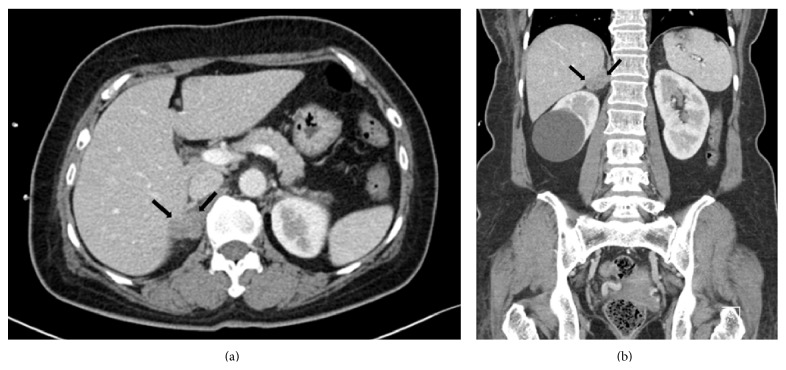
Axial (a) and coronal (b) abdominal computed tomography images during the late arterial phase show a 1.5 cm mass with a lobulated contour and mild enhancement in the right adrenal gland (black arrow).
